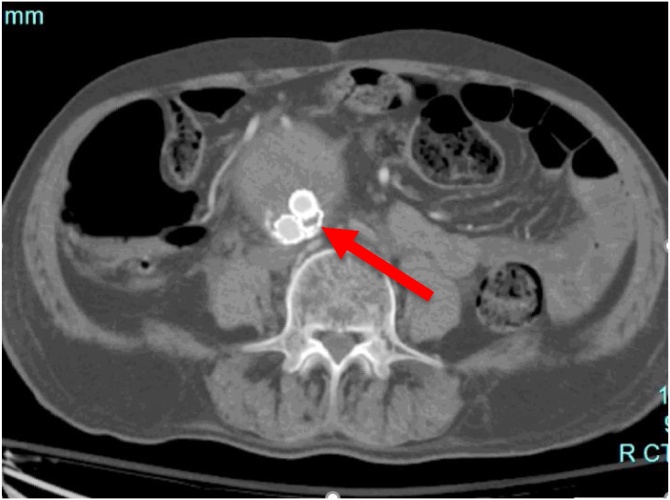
CTA follow up prior to hospital discharge showing the crushed maldeployed stent (RED arrow) and the other patent covered stent. No evidence of endoleak.
